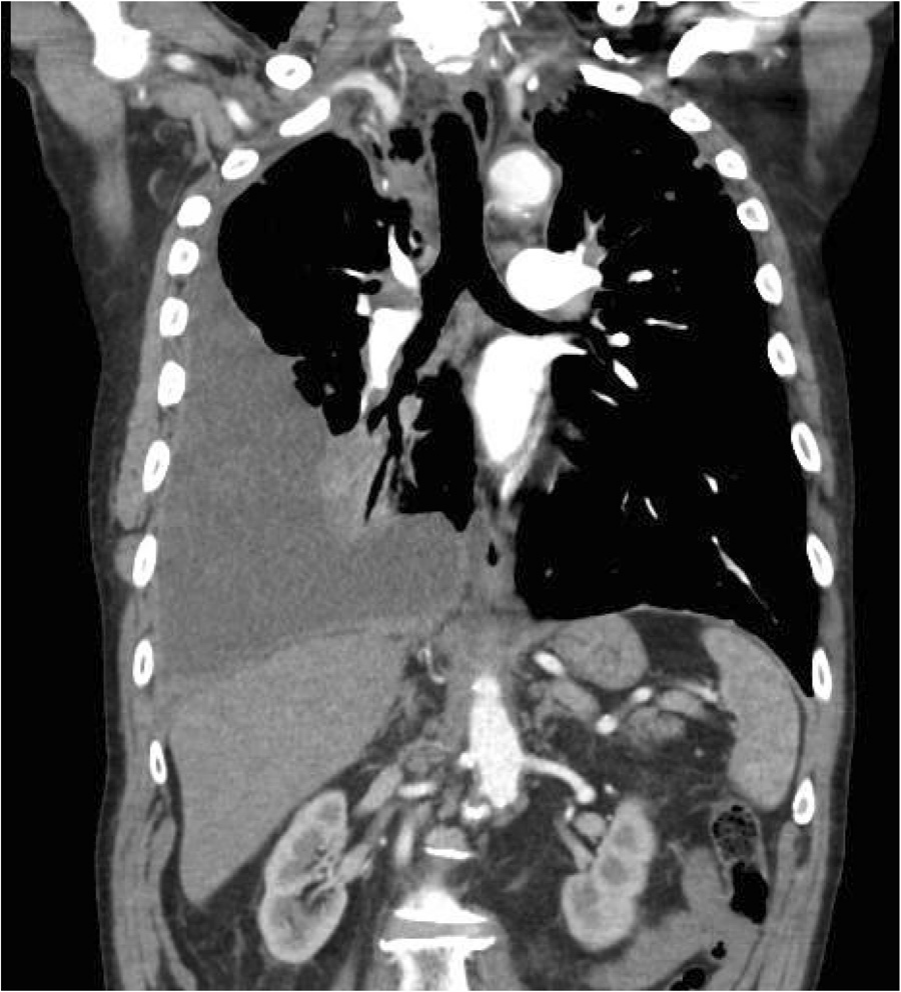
Chest computed tomography scan confirming a large, right-sided pleural effusion in the setting of metastatic papillary thyroid carcinoma, prior to drainage and pleuroscopy.
